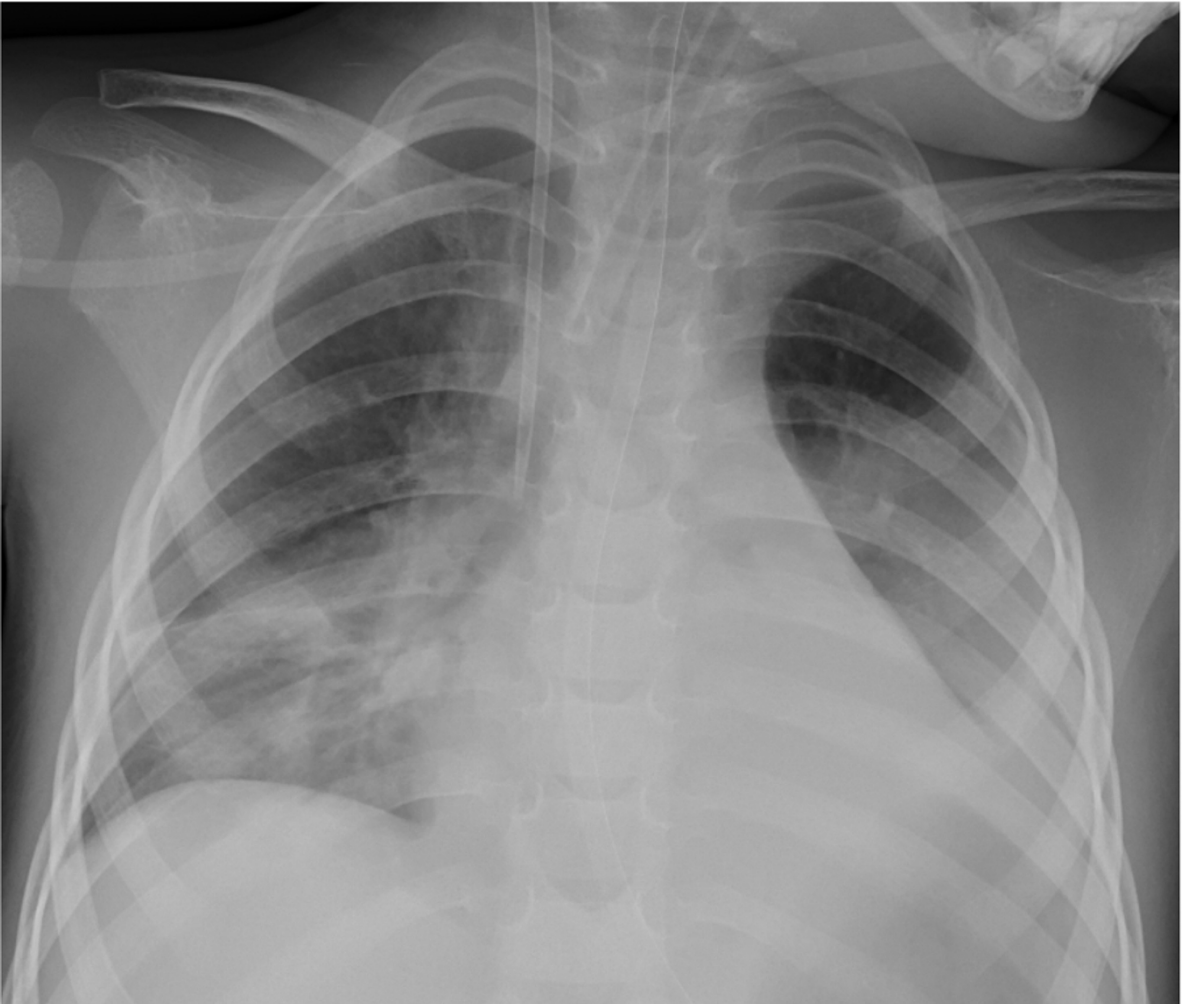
Chest X-ray showed new infiltrations.
